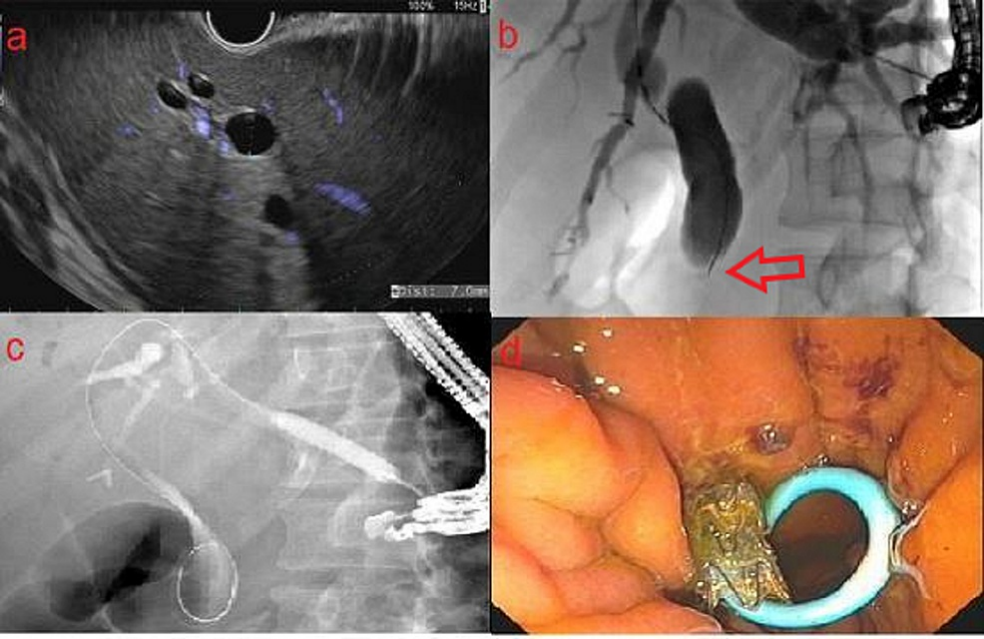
Creation of the hepaticogastrostomy tract using endoscopic ultrasound guidance. a: EUS demonstrating dilated segment 3 biliary radicle. b: Antegrade cholangiogram demonstrating dilated intra and extrahepatic ducts with high grade stricture (red arrow) in the distal common bile duct. c: Dilation of hepaticogastrostomy tract using a Hurricane biliary dilating balloon. d: Successful placement of metal hepaticogastrostomy stent with plastic double pigtail biliary stent anchoring it in place.
